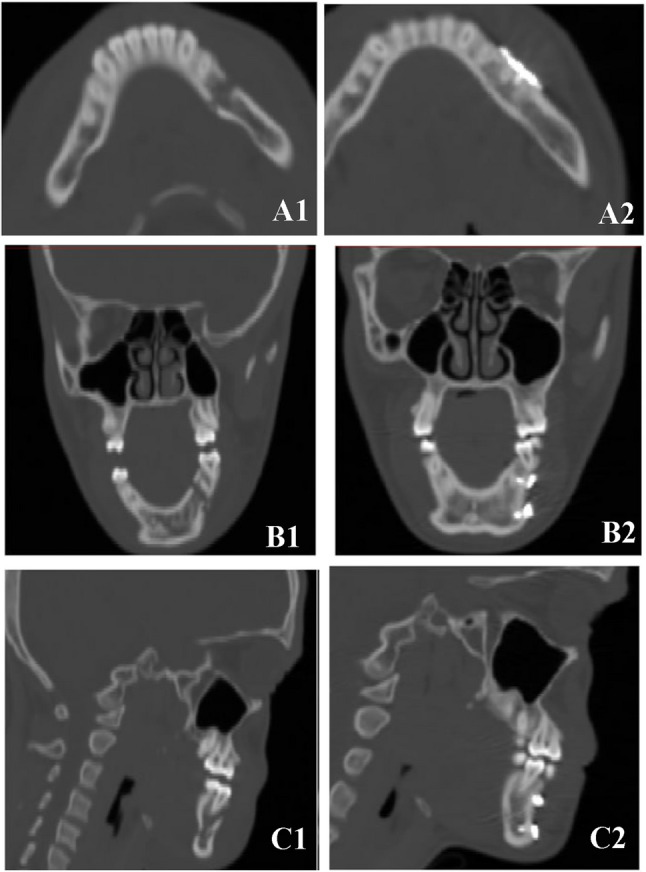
Preoperative vs. postoperative CT images (A1) Preoperative and (A2) Postoperative CT axial cuts. (B1) Preoperative and (B2) Postoperative coronal cuts. (C1) Preoperative and (C2) Postoperative CT sagittal cuts
